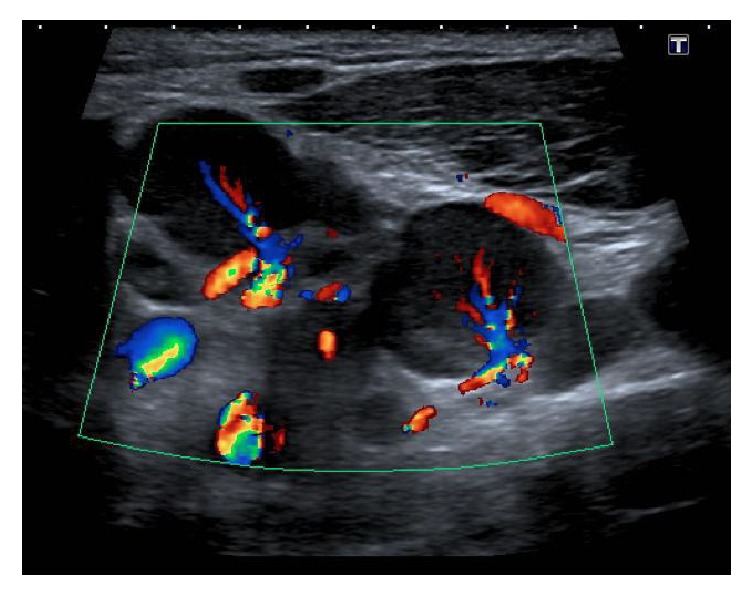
Ultrasound scan showing increased colour Doppler flow through enlarged left cervical lymph nodes.
